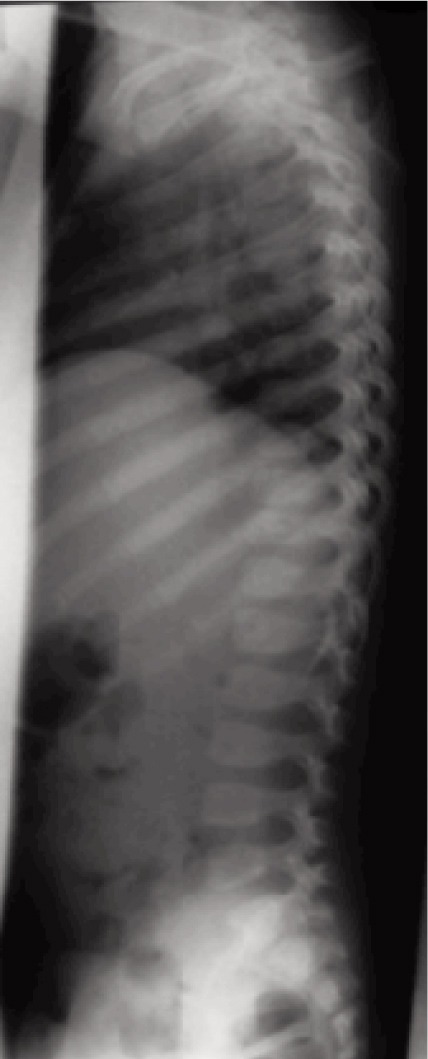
Pre-op lateral radiograph.
